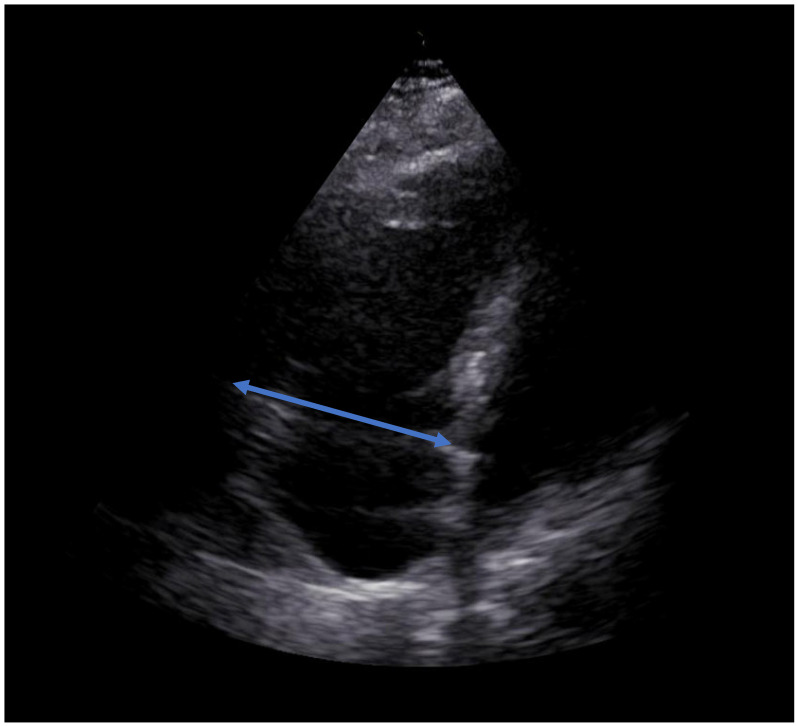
Baseline transthoracic echocardiogram showing a distended right ventricle with a right ventricular end-diastolic diameter (RVEDD) of 6.49 cm (arrow).
